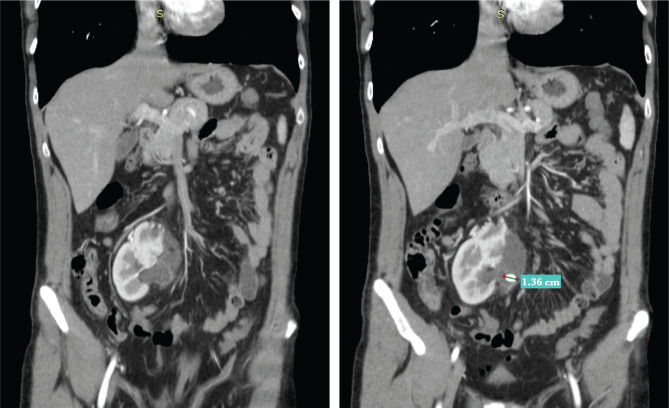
Coronal and sagittal CT sections of one case of ectopic pelvic kidney with duplicity successfully treated through single-use flexible ureteroscopy. The stone measured 1.36 cm on the longest diameter and the stone-free status was obtained after only one intervention.
